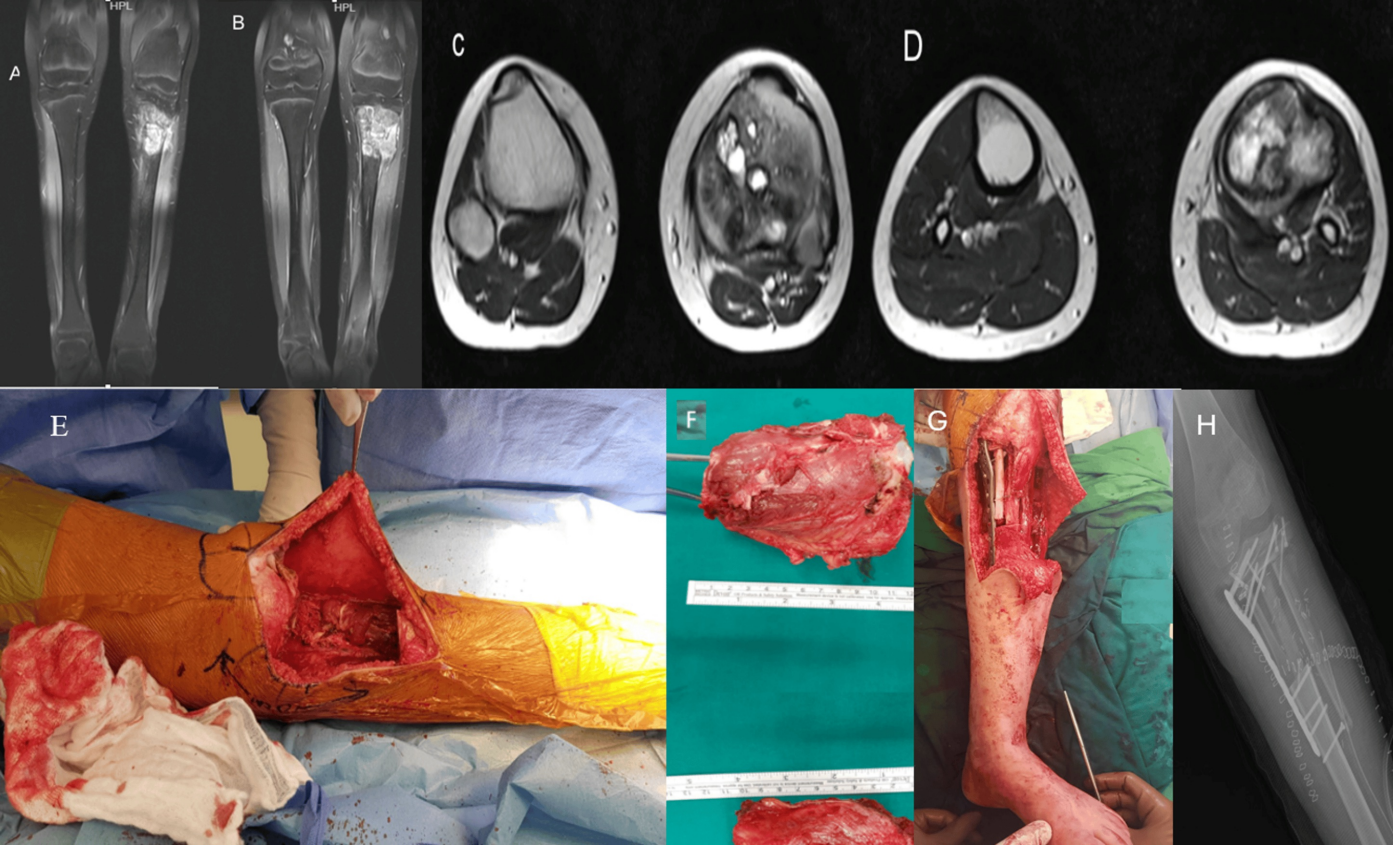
Clinical, imaging, and surgical sequence of a 13-year-old patient with osteoblastic osteosarcoma of the proximal tibia A 13-year-old girl presented with a complaint of swelling below her left knee, persisting for two months. Clinical examination revealed a tender, firm swelling approximately 3x3 cm in size. X-ray findings showed a lytic lesion involving the proximal tibia, raising suspicion of neoplastic involvement. A subsequent biopsy confirmed the diagnosis of osteoblastic osteosarcoma. Following the diagnosis, the patient underwent a course of neoadjuvant chemotherapy comprising Cisplatin, Adriamycin, Doxorubicin, and Methotrexate over six cycles spanning 10 weeks. Post-chemotherapy imaging studies, including MRI, were conducted (Fig A-D). The patient underwent a surgical procedure involving a wide-margin excision and an ipsilateral double-barrel pedicled fibular graft. Intraoperative pictures (Fig E-G) and post-operative X-rays (Fig H) were obtained.
